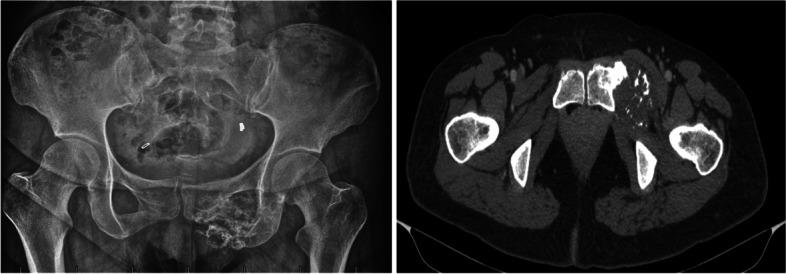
Preoperative images of a 46-year-old chondrosarcoma patient. X-ray (left) shows an irregularly-shaped lesion with calcification in the pubis region. CT scan of the pelvic bone (right) shows a tumor extending from the pubis toward the ischium with intralesional “popcorn-like” calcification
